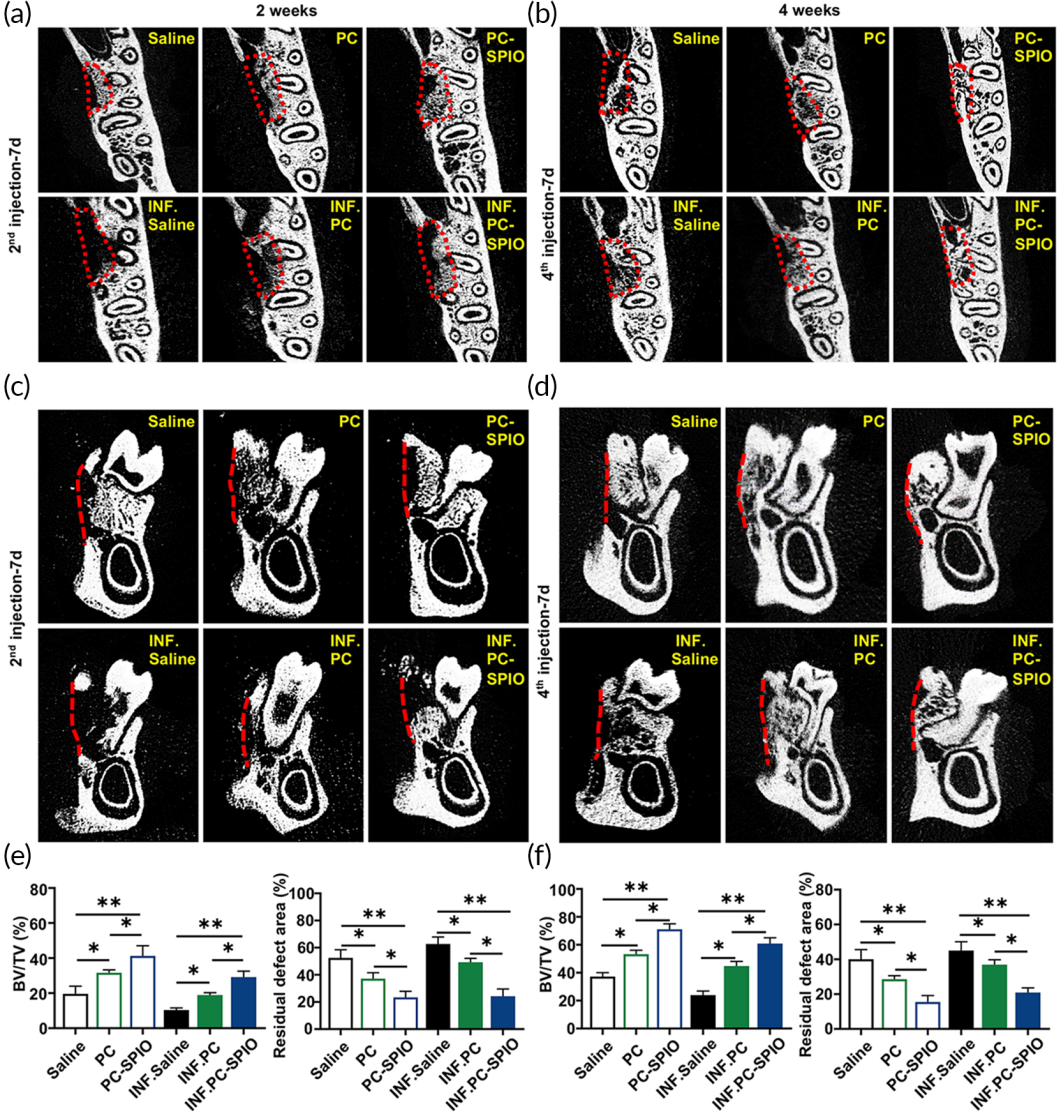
(a) Evaluation of periodontal bone regeneration using micro‐CT at 2 weeks after surgery (transverse planes). (b) Evaluation of periodontal bone regeneration by micro‐CT at 4 weeks after surgery (transverse planes). (c) Evaluation of periodontal bone regeneration using micro‐CT at 2 weeks after surgery (coronary planes). (d) Evaluation of periodontal bone regeneration by micro‐CT at 4 weeks after surgery (coronary planes). (e) Quantitative analysis of periodontal bone regeneration by micro‐CT, including BV/TV and the residual defect area, at 2 weeks after surgery. (f) Quantitative analysis of periodontal bone regeneration by micro‐CT, including BV/TV and the residual defect area, at 4 weeks after surgery. (*n* = 3) (p* < 0.05, *p* < 0.01)
